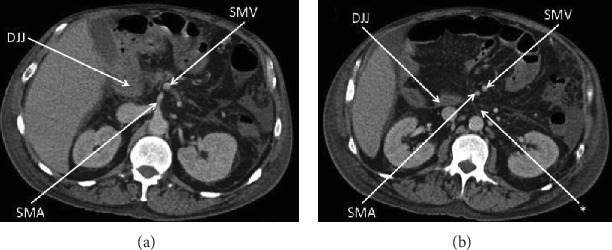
Abdominal computed tomography four years prior to current hospital presentation with incidental classic findings of intestinal malrotation. (a) Transverse plane at the second lumbar vertebral level demonstrating the duodenojejunal junction (DJJ) at the right of the midline and the superior mesenteric vein (SMV) to the left of the superior mesenteric artery (SMA). (b) Transverse plane at the third lumbar vertebral level further demonstrating the duodenojejunal junction (DJJ) at the right of the midline and the superior mesenteric vein (SMV) to the left of the superior mesenteric artery (SMA), as well as the absence of a retroperitoneal third portion of the duodenum (∗).
